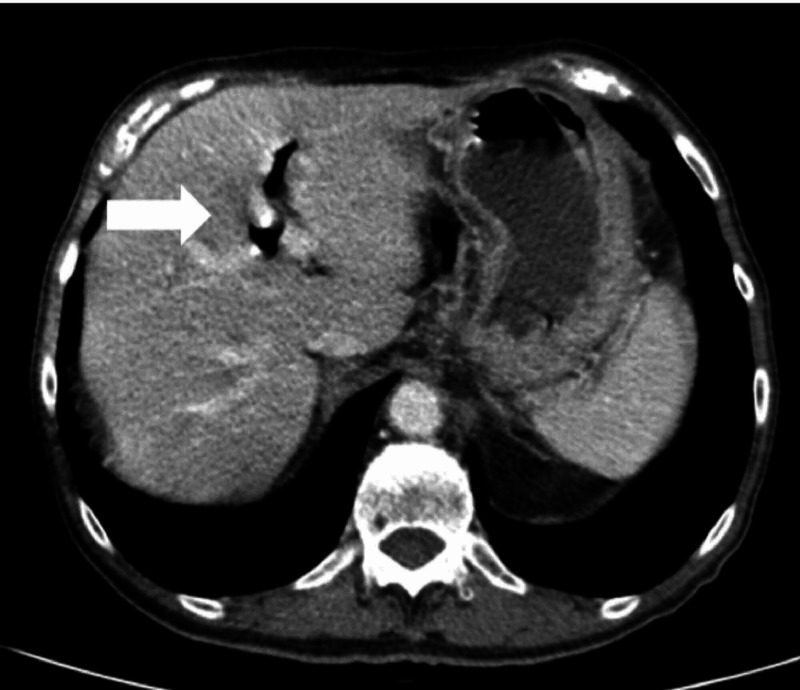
Abdomino-pelvic CT: gastric distension and aerobilia (white arrow).
